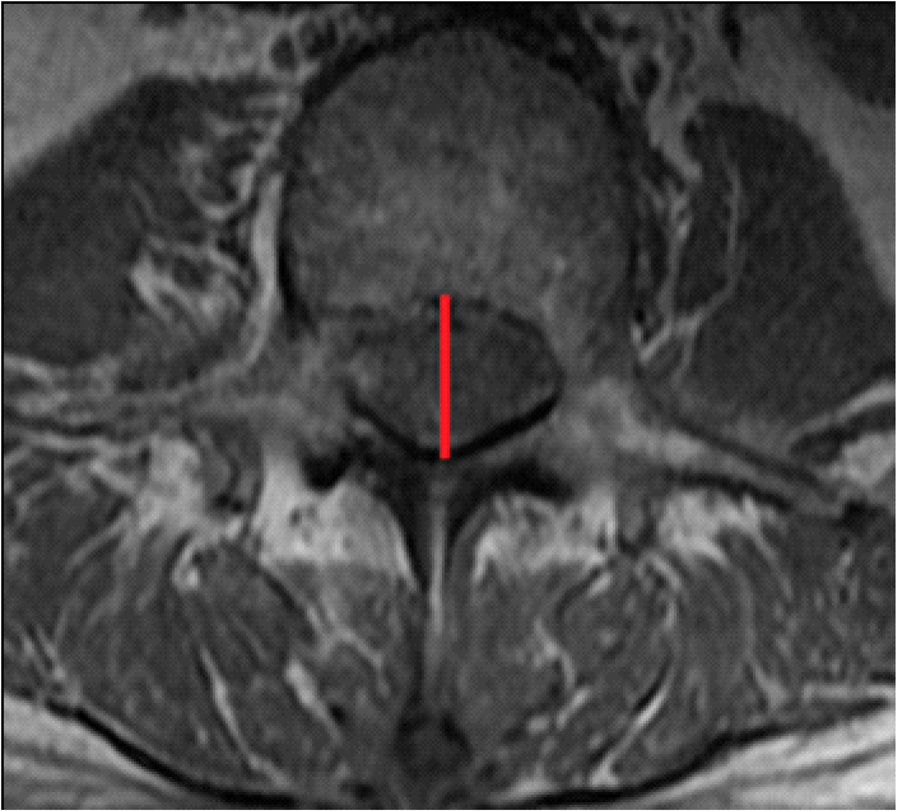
Measurement of midline anteroposterior (AP) spinal canal diameter at L1 to S1 using T1-weighted axial MRI scan (marked in red line) - at the cut where the entire bony canal ring could be seen and with the thickest pedicle width
